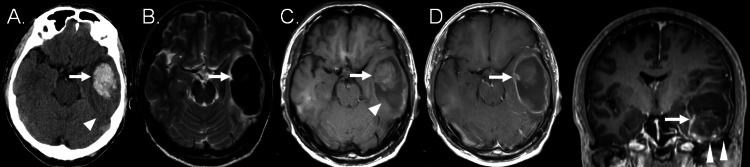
Computed tomography (CT) obtained at the onset and magnetic resonance (MR) images obtained on day five after intracerebral hemorrhage at the left temporal lobe. A: CT, showing mixed hyperdense (arrow) and surrounding isodense lesion (arrowhead) at the left temporal lobe. B–D: MR images, showing a homogenously hypointense lesion on T2-weighted MR image (arrow), (B) mixed isointense (arrowhead), and surrounding hypointense lesion (arrow) on T1-weighted MR image. (C) Peripheral linear and nodular (arrowheads) enhancement around the hematoma and septum (arrows) of the hematoma cavity enhanced on gadolinium-enhanced axial (left panel) and coronal (right panel) T1-weighted MR images. (D).
